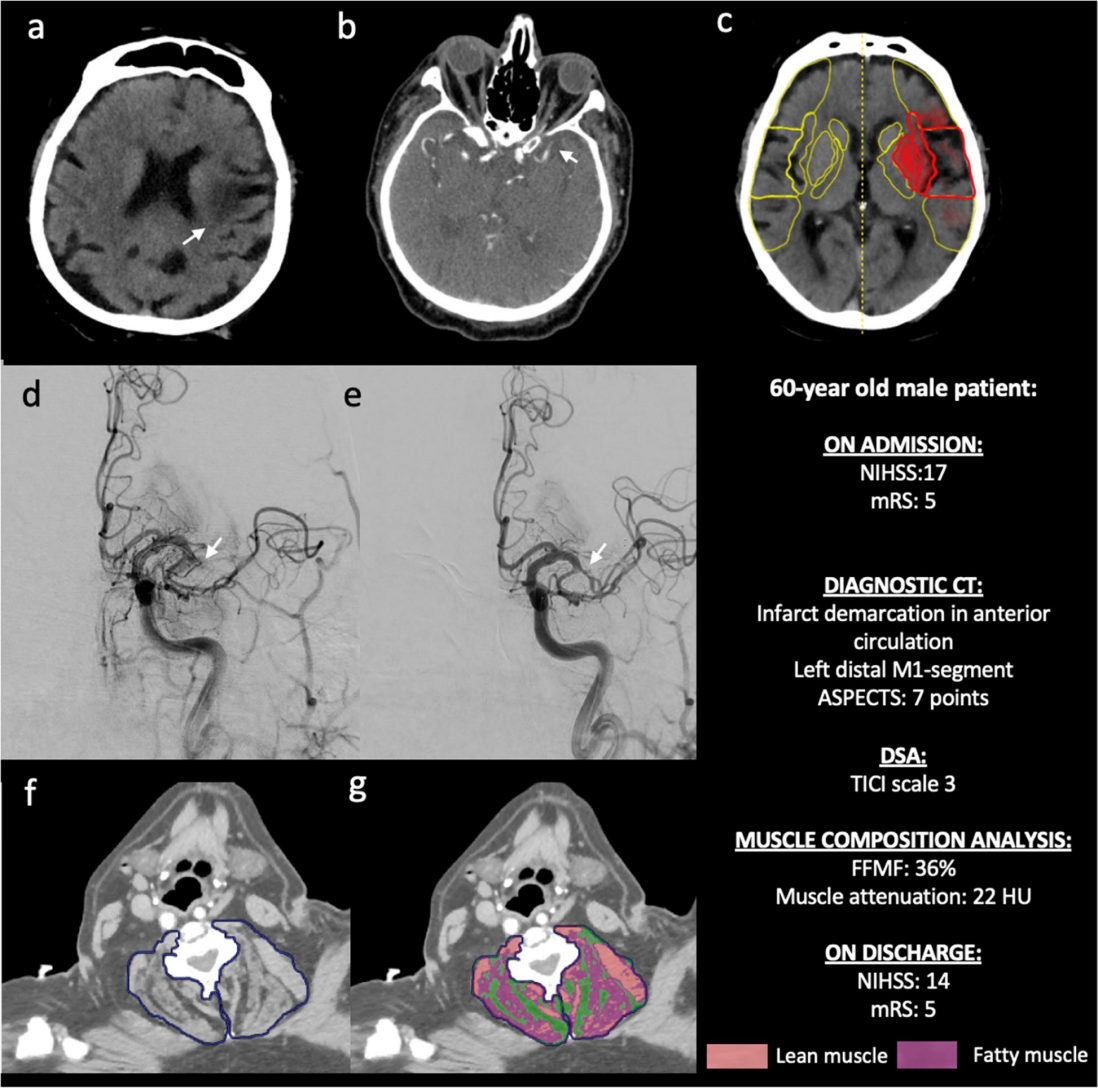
Representative images of a 60-year-old male patient with a low fat-free muscle fraction of 36% and ischemic stroke by left middle cerebral artery occlusion. Non-enhanced CT scan (a) demonstrates beginning infarct area demarcation (white arrow) in the anterior circulation due to occlusion of the distal M1 segment of the left middle cerebral artery (white arrow, b). Additional analysis of computer-assisted early ischemic changes revealed an ASPECT score of 7 (c). The patient underwent systemic thrombolysis and successful endovascular intervention with mechanical thrombectomy and complete recanalization (TICI scale 3) (white arrows, d and e). Images f and g visualize the assessment of skeletal muscle fat infiltration as previously described
